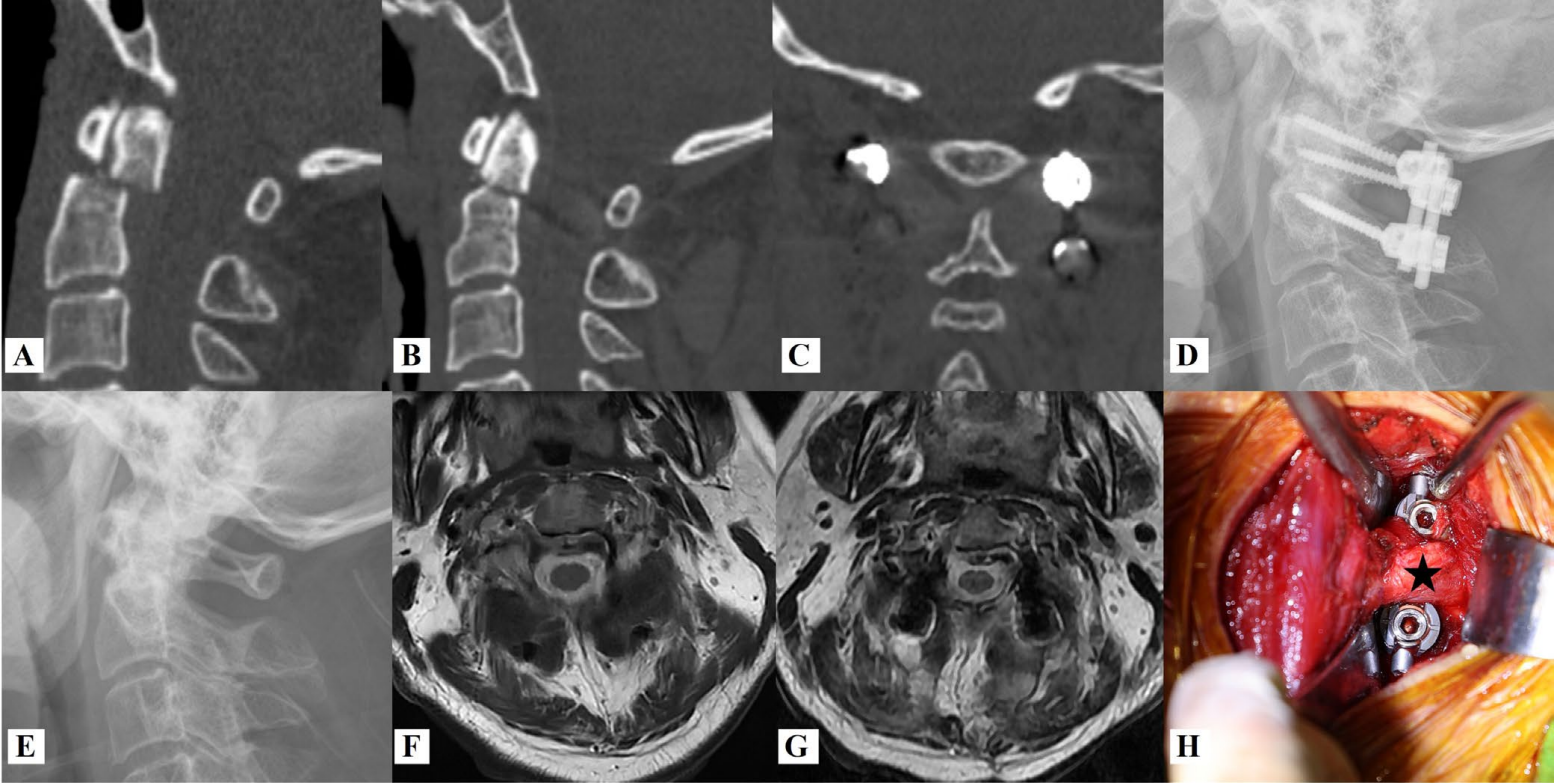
Male, 45 years old, injured in fall accident, Grauer type II_A_ fracture treated by the PMSA. (A) Preoperative sagittal view of CT scan shows odontoid fracture combined with dislocation; (B) postoperative CT scan shows fracture was reset; (C) postoperative coronal view of CT scan shows the preserved signal of the muscles connected to the spinous process of C2; (D) postoperative lateral X‐ray image indicates that the fracture has been successfully realigned; (E) lateral X‐ray imaging of the upper cervical spine following the removal of the internal fixator revealed signs of odontoid healing; (F, G) axial views of MRI before surgery and 3rd days post‐operation; (H) posterior view of the surgical site after locking the screw‐rod system, the black star indicates the OCI.
